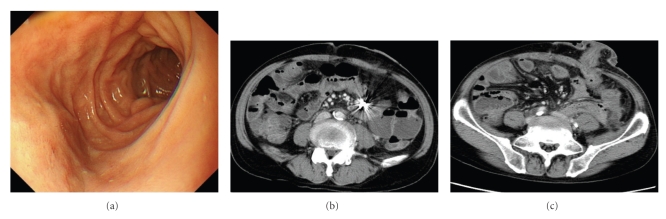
(a) Upper endoscopy on day 14 post coil embolization reveals neither ulceration nor stenosis of the third portion of the duodenum. (b and c) Computed tomography on day 14 post coil embolization. Intraabdominal abscess formation is not observed around the microcoils (b). The end colostomy was edematous and significantly affected by the obliteration of the left colic artery (c).
